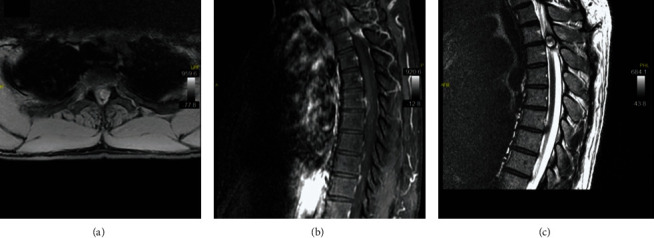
(a) Axial T1-weighted MRI, (b) sagittal T1-weighted MRI, and (c) sagittal T2-weighted and short tau inverted recover MRI images demonstrating a large left T2-T3 synovial facet cyst within the spinal canal.
